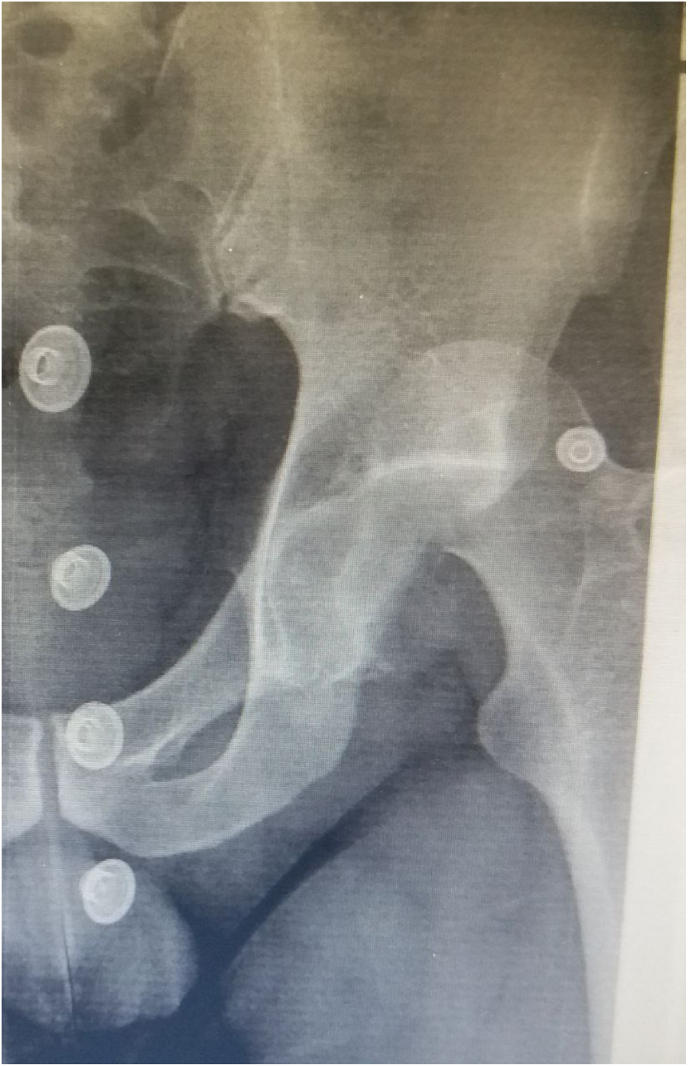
AP Preoperative X-ray of the pelvis shows the dislocation of the left hip joint A Computerized tomography (CT) scan of the hip showed a large femoral head fragment inferior to the fovea centralis (Fig. 2).
